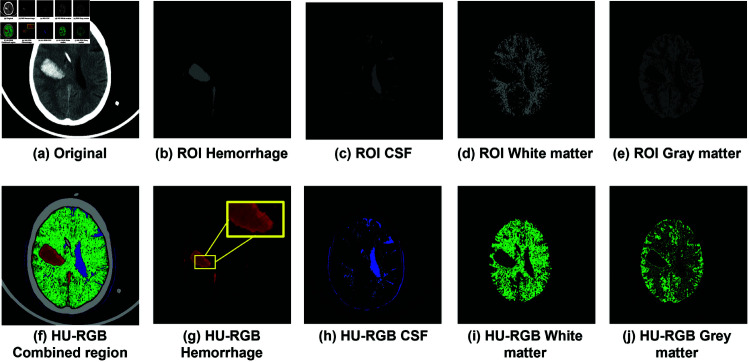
(a)–(e) shows ROI of each component obtained from the proposed HRT method. (a) An original CT, (b) Image of hemorrhage region $I_{\hat{H}}$, (c) Image of CSF $I_{\hat{C}}$, (d) Image of white matter $I_{\hat{M}}$, and (e) Image of gray matter $I_{\hat{G}}$. (f) - (j) depicts the result of the RGB mapped result usnig our proposed method.
